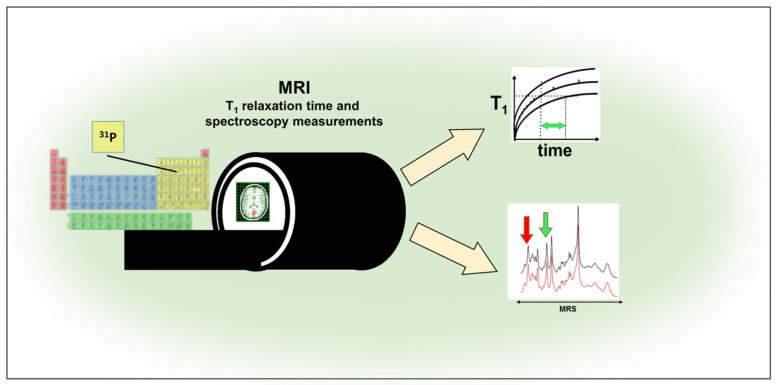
Application Range of ^31^P MRI.
